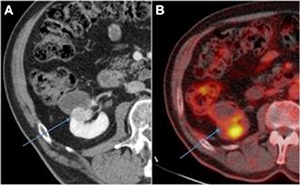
Local RCC recurrence of RM-CA. At 8 months following LCA for patient number 6, a focal contrast-enhanced area at the posterior side within the ablation zone was noticed on contrast-enhanced CT scan (A, blue arrow). Although not as sharply distinctive as the contrast-enhanced CT scan, we noticed metabolic activation in the same posterior rim area at 18F-FDG PET-CT scan (B, blue arrow).
